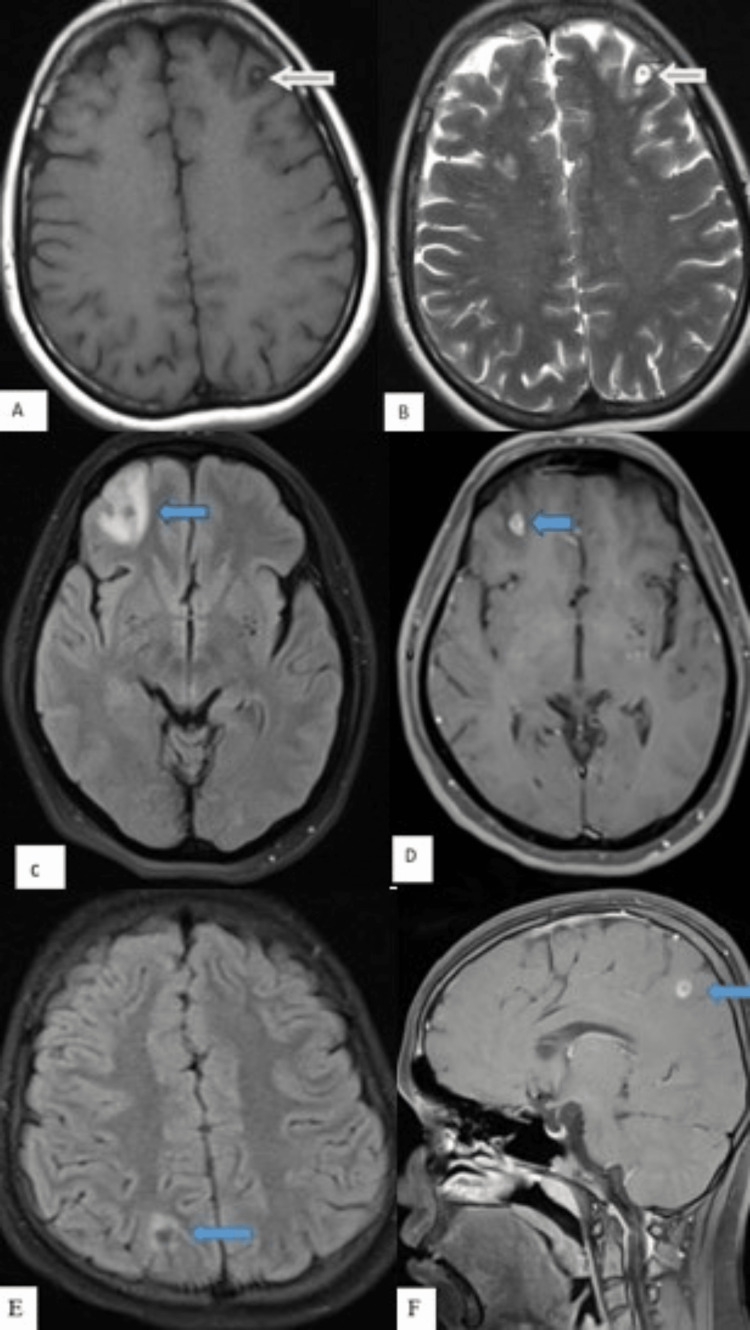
Stages of neurocysticercosis (A-B) Vesicular stage of neurocysticercosis. (A) Axial T1WI and (B) axial T2WI. These images show a well-defined, CSF intensity lesion (orange arrow) demonstrating a central T1 hyperintense dot sign in the left frontal lobe. (C-D) Colloidal vesicular stage of neurocysticercosis. (C) Axial FLAIR and (D) axial T1-weighted post-contrast images. These images show a well-defined T2 hypointense focus (measuring 6 mm) (blue arrows) in the right frontal lobe with mild perilesional edema. The lesion shows ring enhancement in the post-contrast study. (E-F) Granular nodular stage of neurocysticercosis. (E) Axial FLAIR and (F) axial T1-weighted post-contrast images. These images show a FLAIR hypointense lesion with minimal perilesional edema (blue arrows) noted in the right parietal lobe demonstrating peripheral rim enhancement in the post-contrast study. T1WI: T1-weighted imaging; T2WI: T2-weighted imaging; FLAIR: fluid-attenuated inversion recovery.
